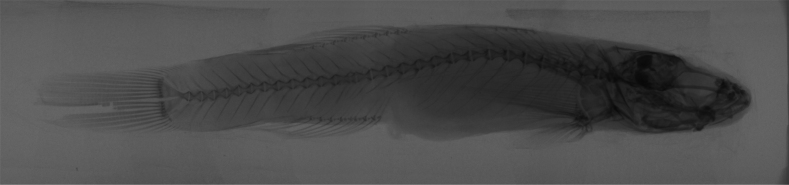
The skeletal system of *R.lithopolychroma* sp. nov. Radiograph graphs of the whole body for paratype RL20230201, females.
